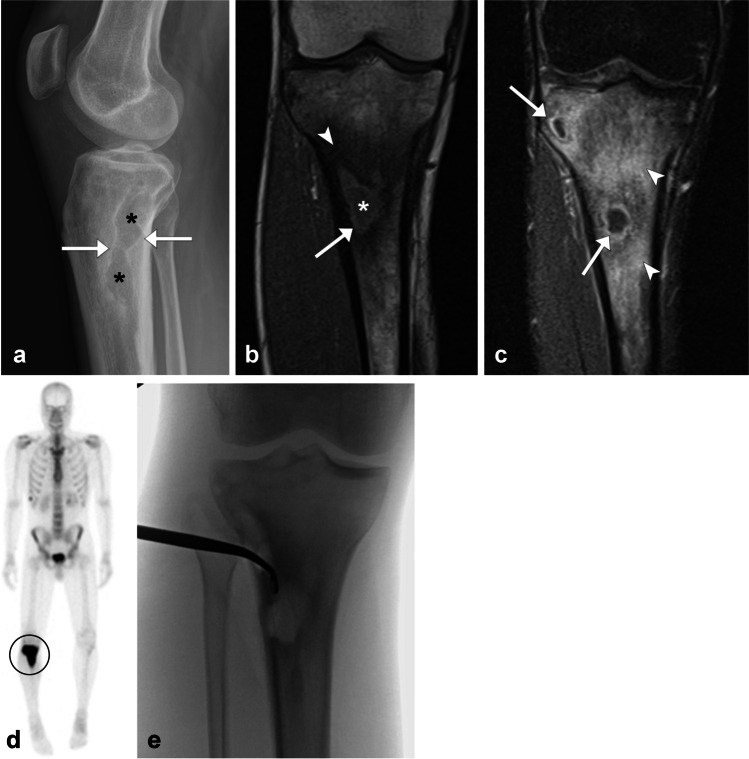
Twenty-seven-year-old man with chronic hematogenous OM. Lateral radiograph of the knee (a) demonstrates an irregular area of lucent bone (asterisks) with surrounding reactive sclerosis (arrows). Coronal T1-weighted MR image (b) of the proximal tibia shows an intraosseous abscess (asterisk) with hyperintense rim or penumbra sign (arrow) and surrounding reactive sclerosis (arrowhead). Coronal T1 postcontrast fat saturated MR image (c) highlights additional smaller abscesses with peripheral enhancement (arrows) and extensive surrounding bone marrow enhancement (arrowheads). Whole body bone scan (d) demonstrates intense uptake in the proximal right tibia (circle). Fluoroscopy image (e) was performed intraoperatively at which time approximately 20 mL of purulent material was aspirated from three pockets. Frozen section showed a mixed inflammatory infiltrate
